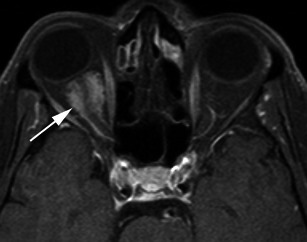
Post-contrast axial T1-weighted sequence in a 64-year-old man with proptosis demonstrates a homogeneously enhancing, intraconal mass within the right orbit (*arrow*) that partially encircles the right optic nerve. Smooth dural thickening and enhancement extends posteriorly to the optic canal. The imaging appearance is consistent with a meningioma
